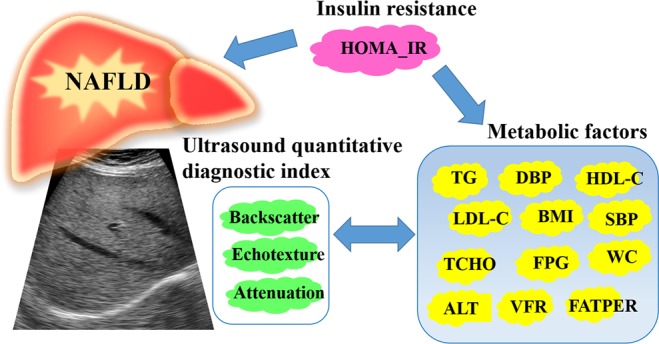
Interrelationship between metabolic syndrome and ultrasound quantitative diagnostic index used for non-alcoholic fatty liver disease (NAFLD) detection. Abbreviations: BMI: body mass index; WC: waist circumference; SBP: systolic blood pressure; DBP: diastolic blood pressure; FATPER: fat percentage; VFR: visceral fat rating; FPG: fasting plasma glucose; TCHO: total cholesterol; TG: triglycerides; HDL-C: high-density lipoprotein cholesterol; LDL-C: low-density lipoprotein cholesterol; ALT: alanine aminotransferase; HOMA-IR: homeostasis model assessment of insulin resistance.
